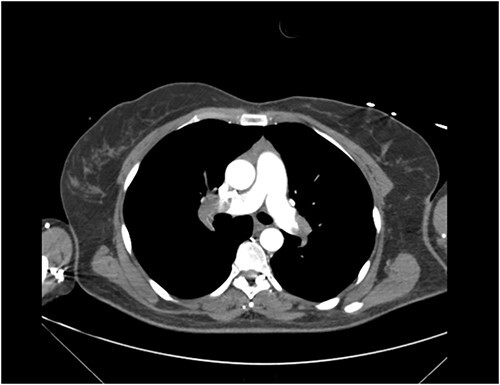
CT Pulmonary Angiogram demonstrating filling defects in the left and right pulmonary arteries.
